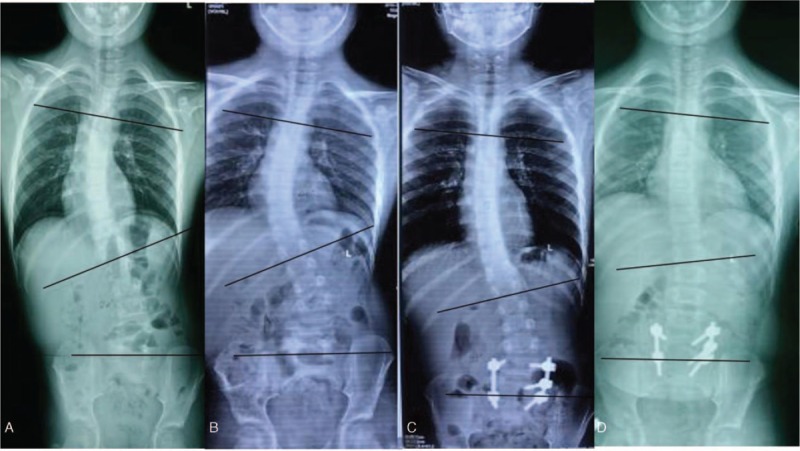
A, Spine X-ray in 2014.11: T5-T12 Cobb: 36°, L1-L5: 20°. B, Spine X-ray in 2015.12: T5-T12 Cobb: 40°, L1-L5: 27°. C, Spine X-ray 1 week after surgery: T5-T12 Cobb: 15°, L1-L5: 20°. D, Spine X-ray 3 months after surgery: T5-T12 Cobb: 10°, L1-L5: 12°.
